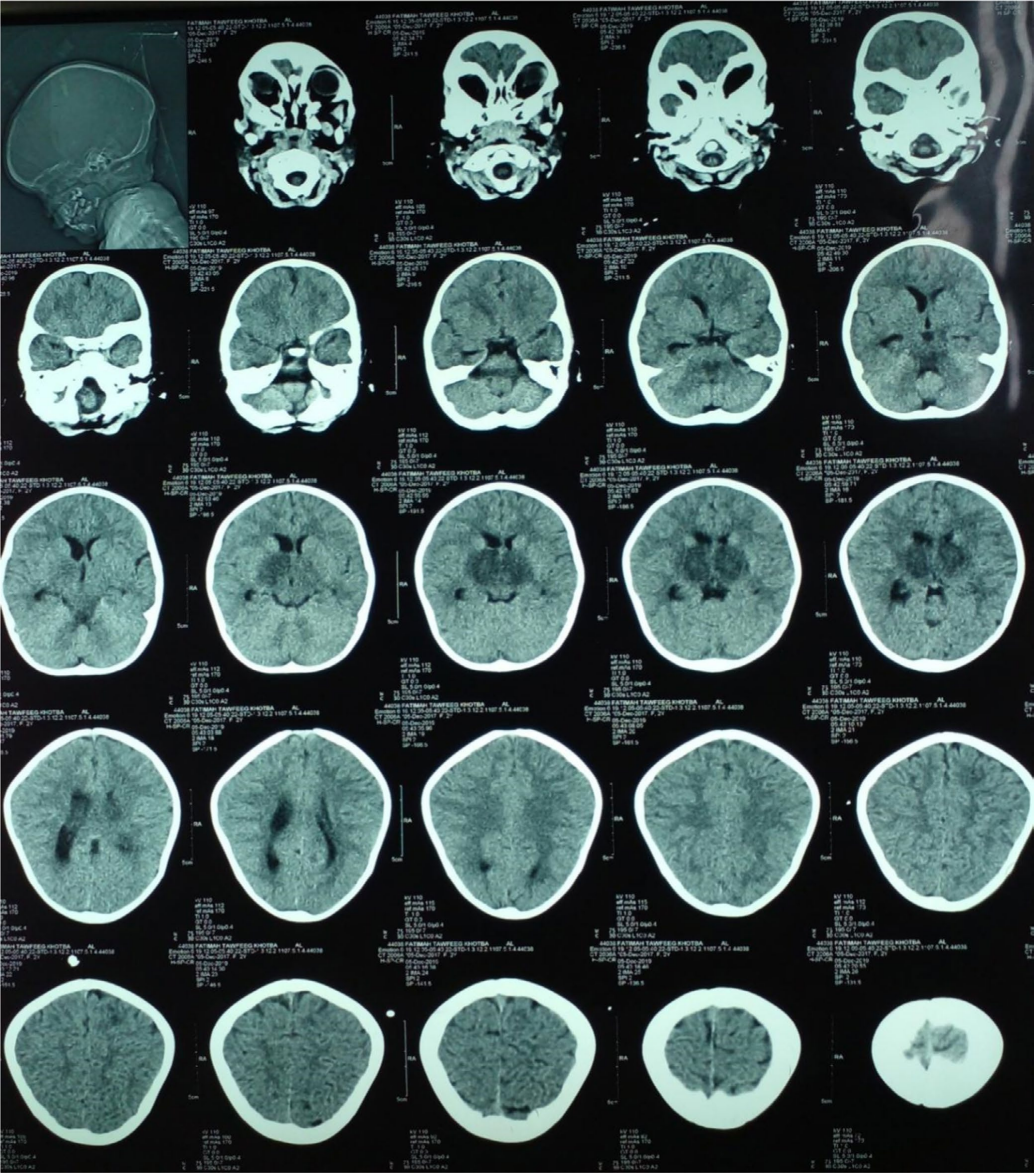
CT of the brain was done which revealed bilateral basal ganglia infarction
